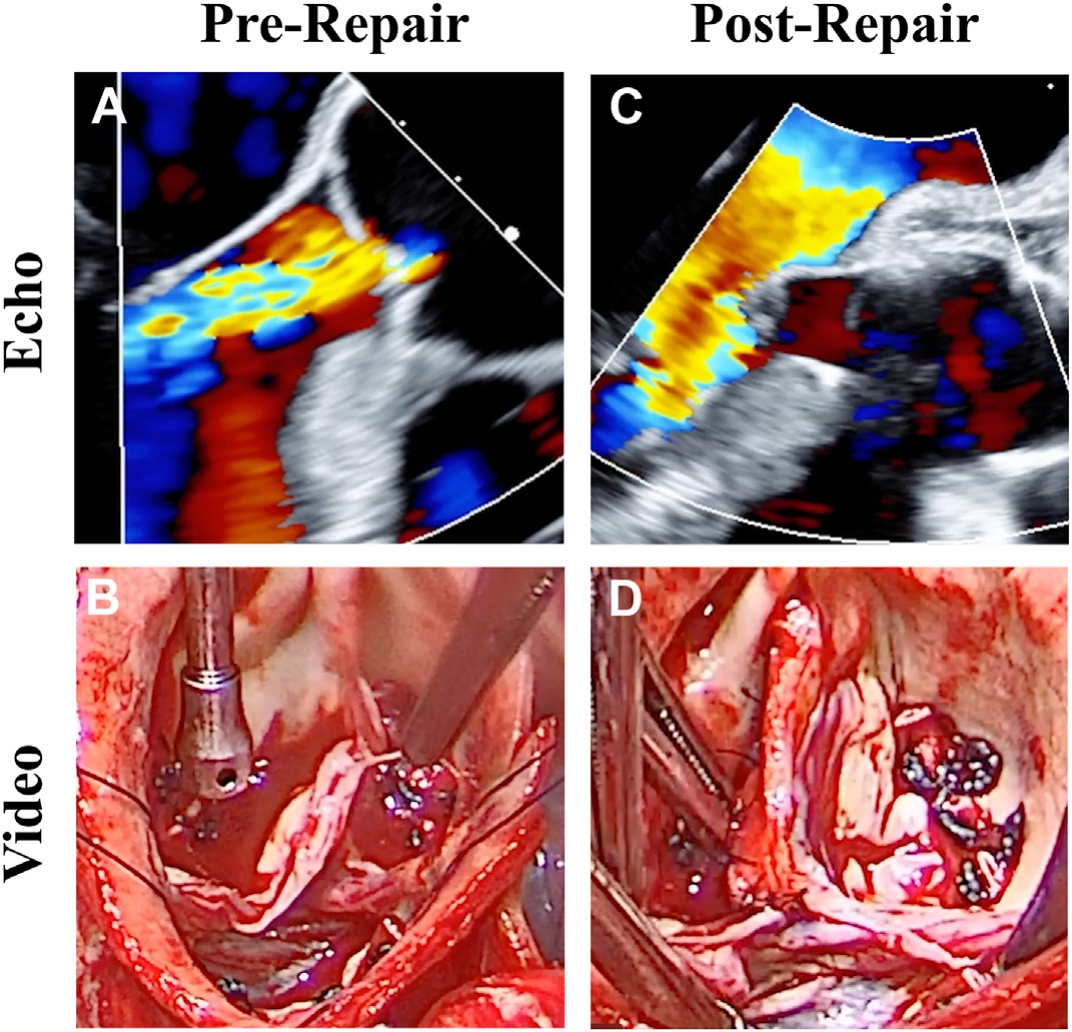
Echocardiographic and video views of valve for patient 2 showing severe posterior aortic insufficiency (A) from ruptured right coronary fenestration (B). After repair, valve was fully competent (C) with strip of aortic wall sutured into the right leaflet and commissure (D).
